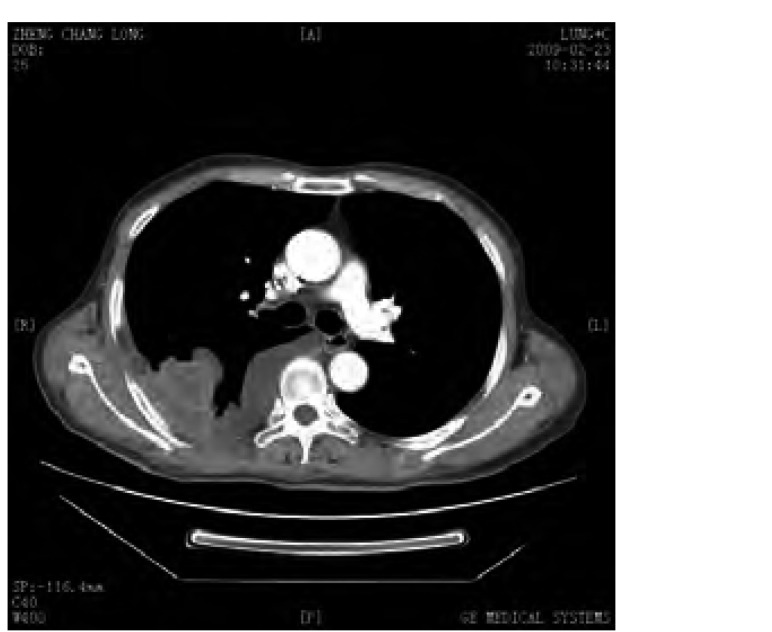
患者确诊时的CT显像。右肺原发灶，右胸膜转移癌，右胸腔积液 CT image of definite diagnosis. Right lung cancer, Right pleural metastasis, and Right pleural effusion
